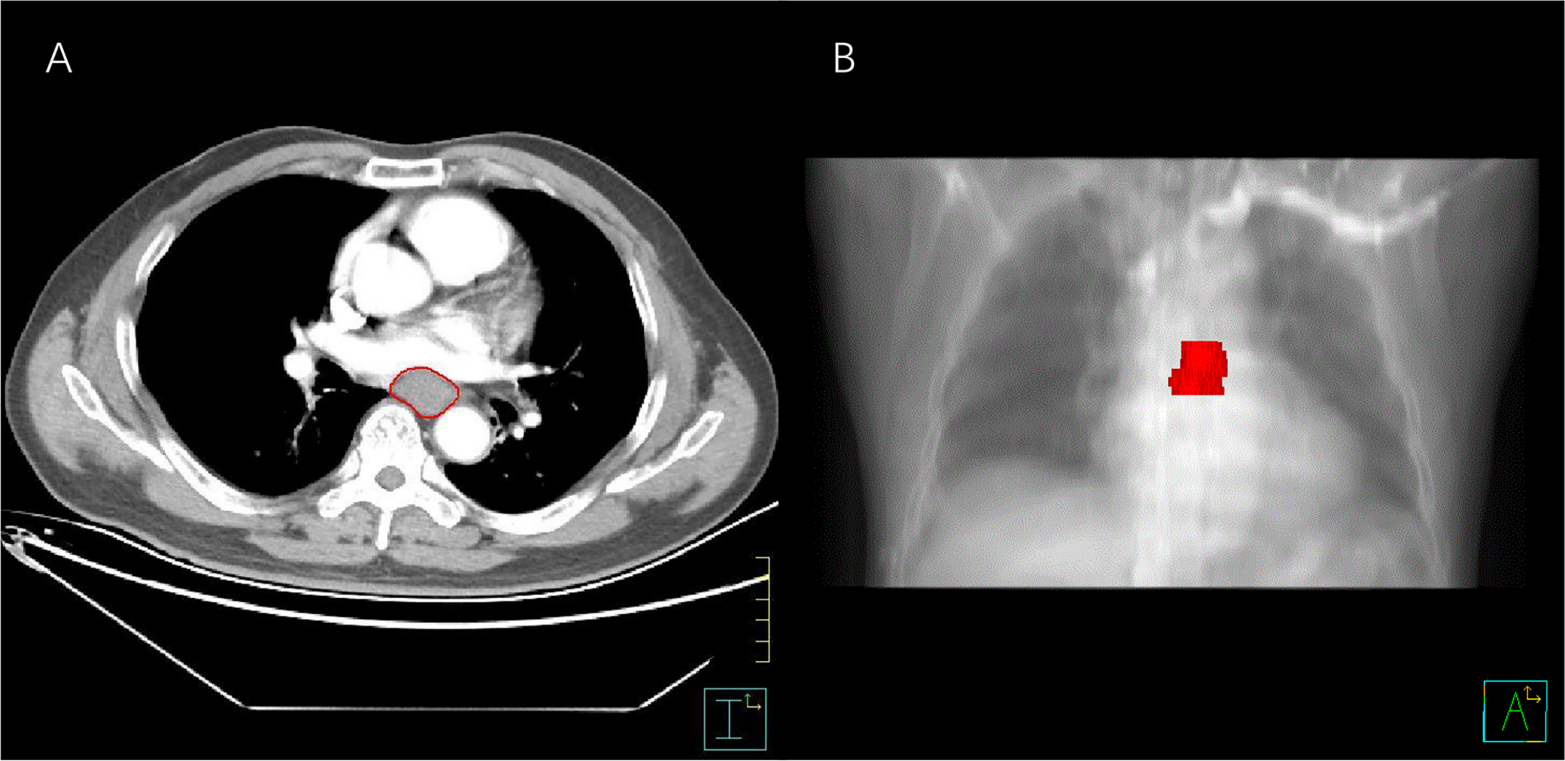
The process of segmentation. (A) VOI contouring on an enhanced axial CT slice. (B) Three-dimensional image of esophageal cancer. VOI, volume of interest.
